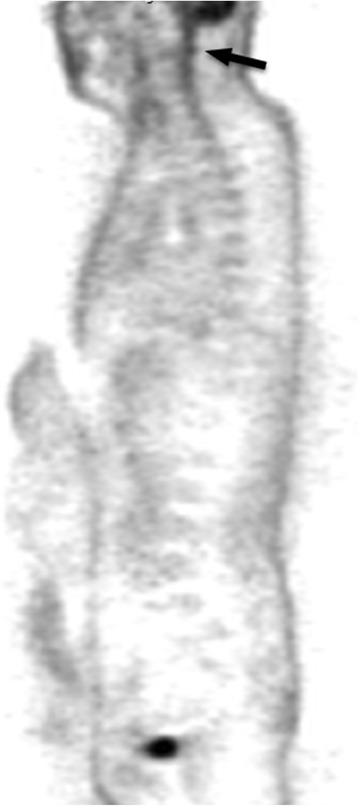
Half-body FDG-PET showing intense uptake along the brainstem, cerebellum, cervical and thoracical spinal cord (*arrow*)
